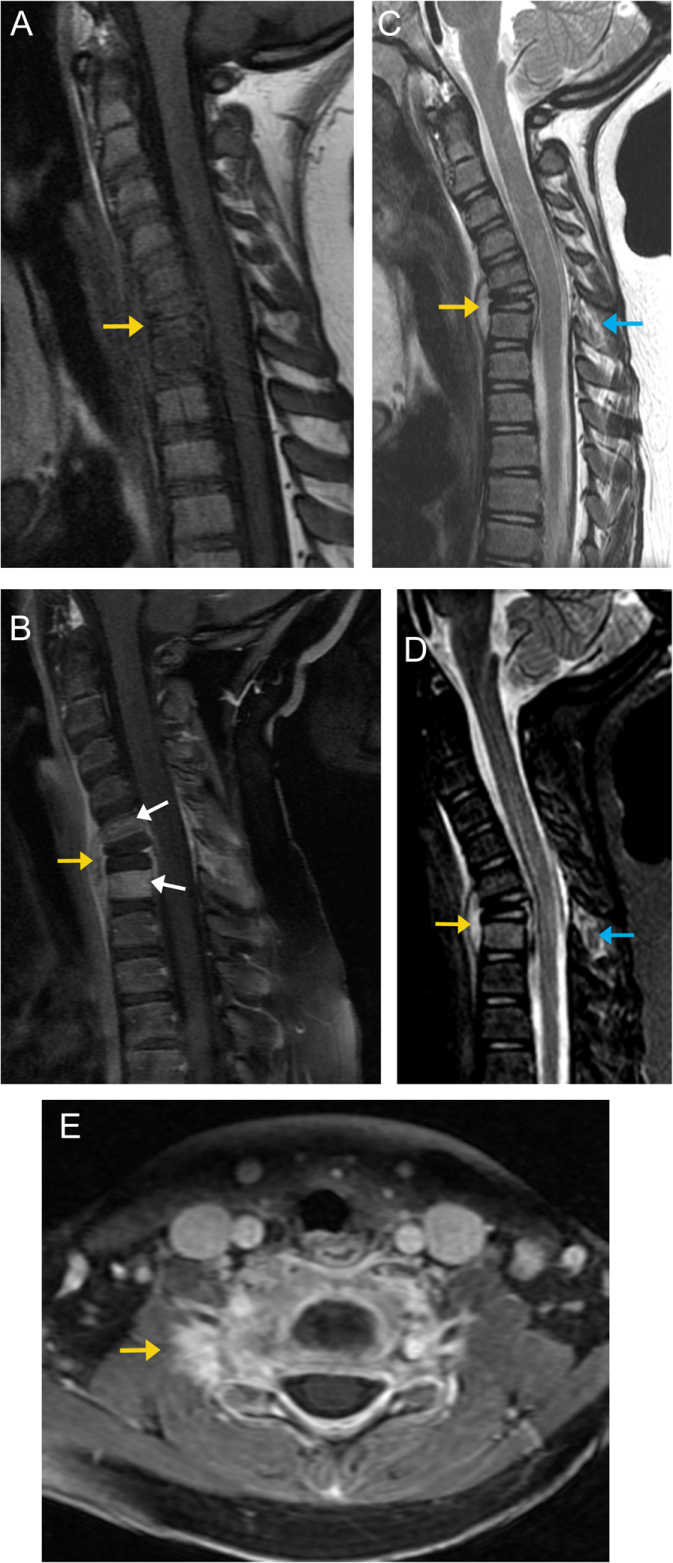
Eosinophilic granuloma. Sagittal T1 image (a) demonstrates suggestion of vertebral plana (orange arrow), however difficult to detect due to the lesion appearing isointense to pediatric marrow on T1. Sagittal T1 post-contrast MR image (b) shows an enhancing mass (orange arrows) with diffuse marrow infiltration of C5 and C7 (white arrows), and C6 pathologic vertebral collapse, sparing the adjacent vertebral endplates and discs. Sagittal T2 (c) and T2 fat -saturated (d) images demonstrate the lesion as a heterogeneously hyperintense soft tissue mass. There is edema of the posterior interspinous soft tissue, more evident on fat suppressed image (blue arrows). Axial T1 post contrast image (e) demonstrates asymmetric enhancement surrounding the right facets (orange arrow)
